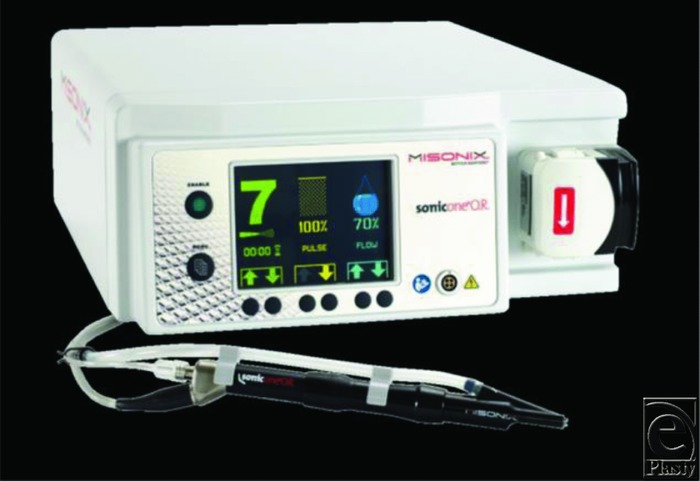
SonicOne ultrasonic debridement system combines aspiration with ultrasonic debridement.
